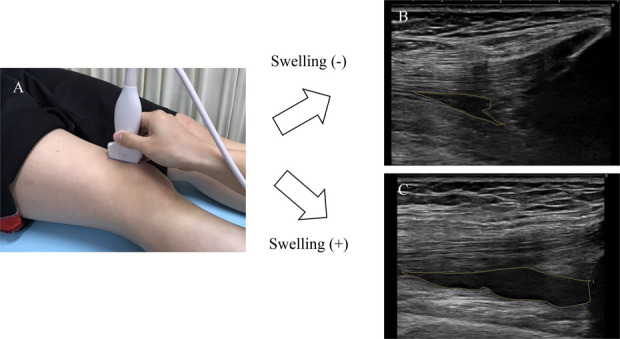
Quantitative evaluation of effusion in the suprapatellar bursa (objective swelling). (A) The ultrasound scan image of the suprapatellar bursa was acquired by placing a linear probe longitudinally on the suprapatellar pouch. During the examination, the transducers were placed as gently as possible, as pressure on the skin through the transducers can affect the acquired effusion area. Representative images of the suprapatellar swelling area: 30 mm^2^ (B); 100 mm^2^ (C).
